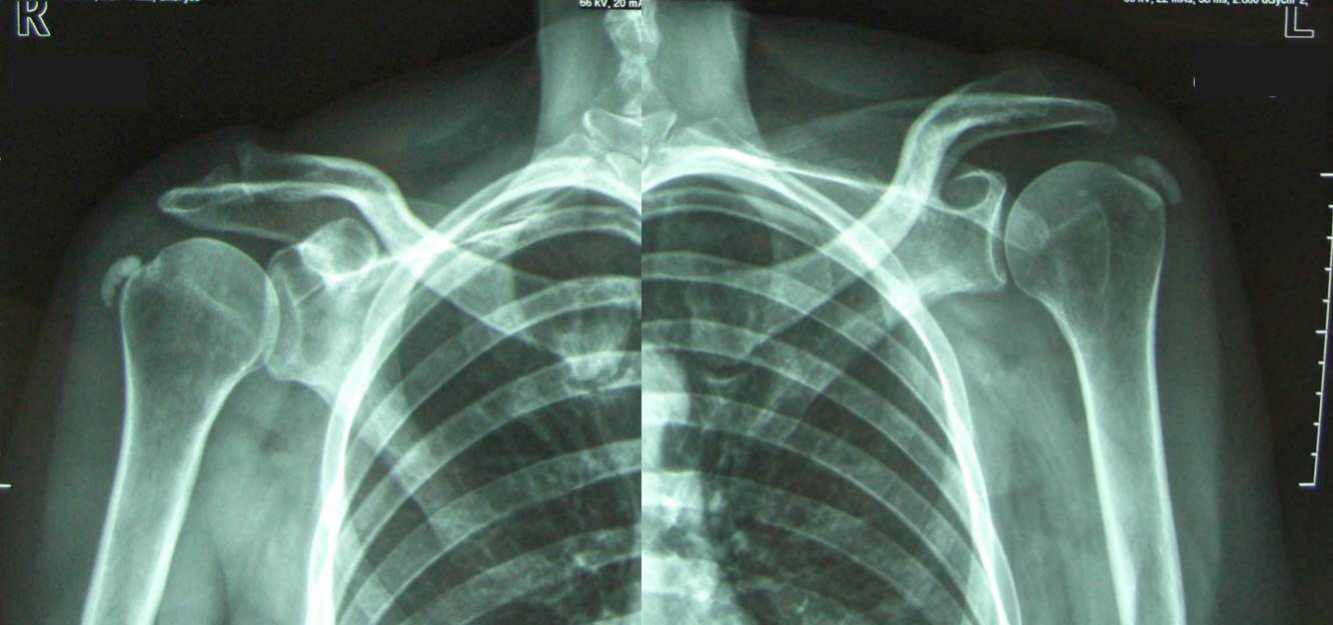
Bilateral calcific tendinopathy (CT) of the shoulder.
